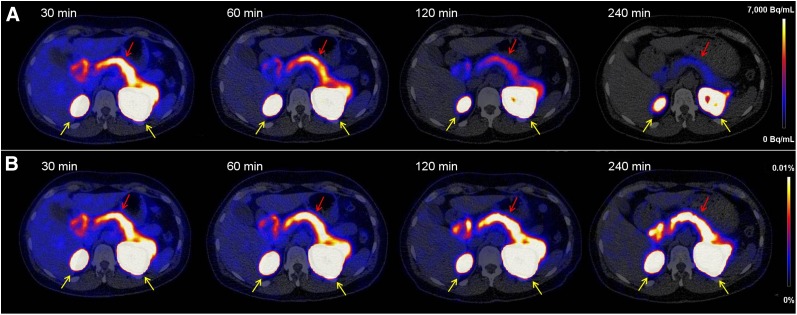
Transversal PET/CT images of abdomen showing biodistribution of ^68^Ga-NODAGA-exendin-4 at 30, 60, 120, and 240 min after injection. (A) Tracer accumulation in Bq/mL, showing washout as well as physical decay. (B) Tracer accumulation in percentage injected dose/g of tissue, showing only washout of tracer. Kidneys are indicated by yellow arrows and pancreas by red arrows.
